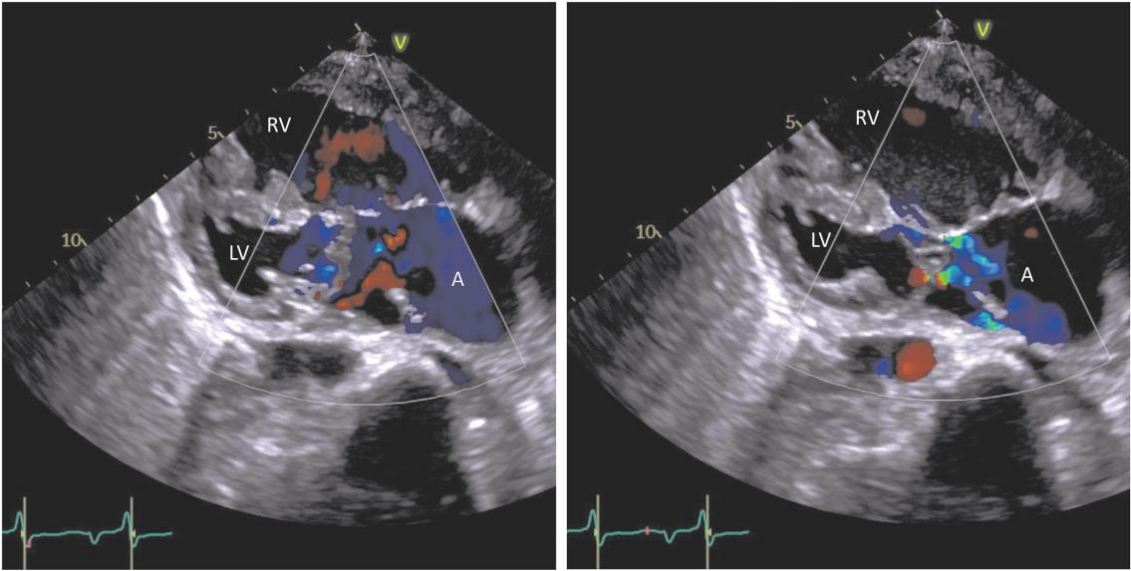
Modified parasternal long-axis view of the systemic right ventricle (RV), hypoplastic left ventricle (LV), atrial complex (A), and atrioventricular valves, demonstrating end-diastolic (left) and end-systolic (right) dimensions and AV-regurgitation on arrival at the tertiary care centre.
